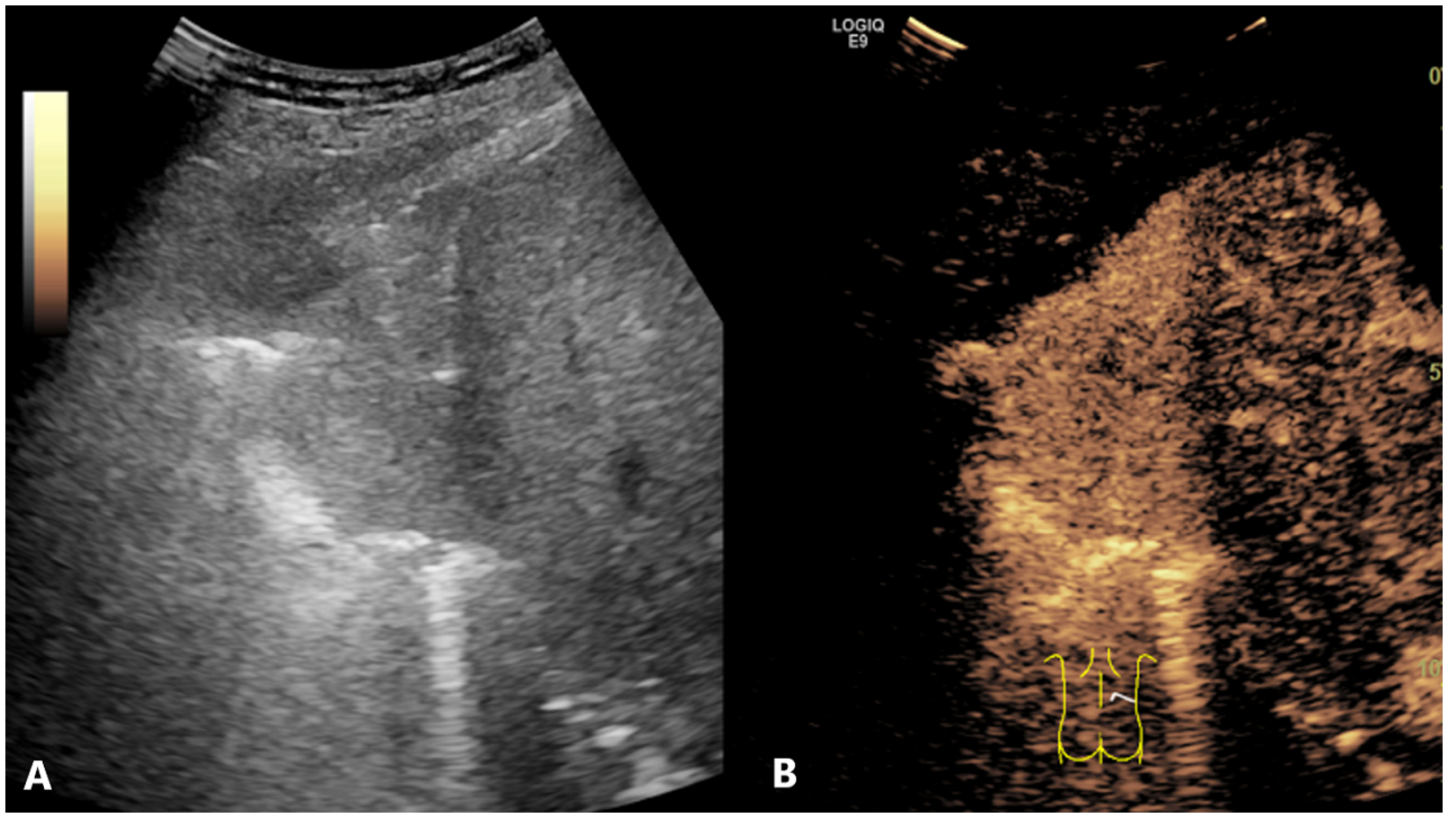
Conventional US and CEUS images of a subpleural pulmonary lesion (LUAD) in the lower lobe of right lung of a 64-year-old female. Conventional US (A) revealed an isoechoic lesion with a maximum diameter of 6.3 cm. The lesion exhibited an irregular shape and a homogeneous composition. CEUS (B) displayed a homogeneous pattern of hyper-enhancement in the lesions, accompanied by the absence of perfusion defects. The serum CEA result was negative. The probability of LUSC, as calculated by the prediction model, was approximately 0.1, which was less than the cutoff value (0.375). This indicated that the lesion was predicted to be LUAD, which was consistent with the definitive diagnosis. US, ultrasound; CEUS, contrast-enhanced ultrasound; LUAD, lung adenocarcinoma; LUSC, lung squamous cell carcinoma; CEA, carcinoembryonic antigen.
